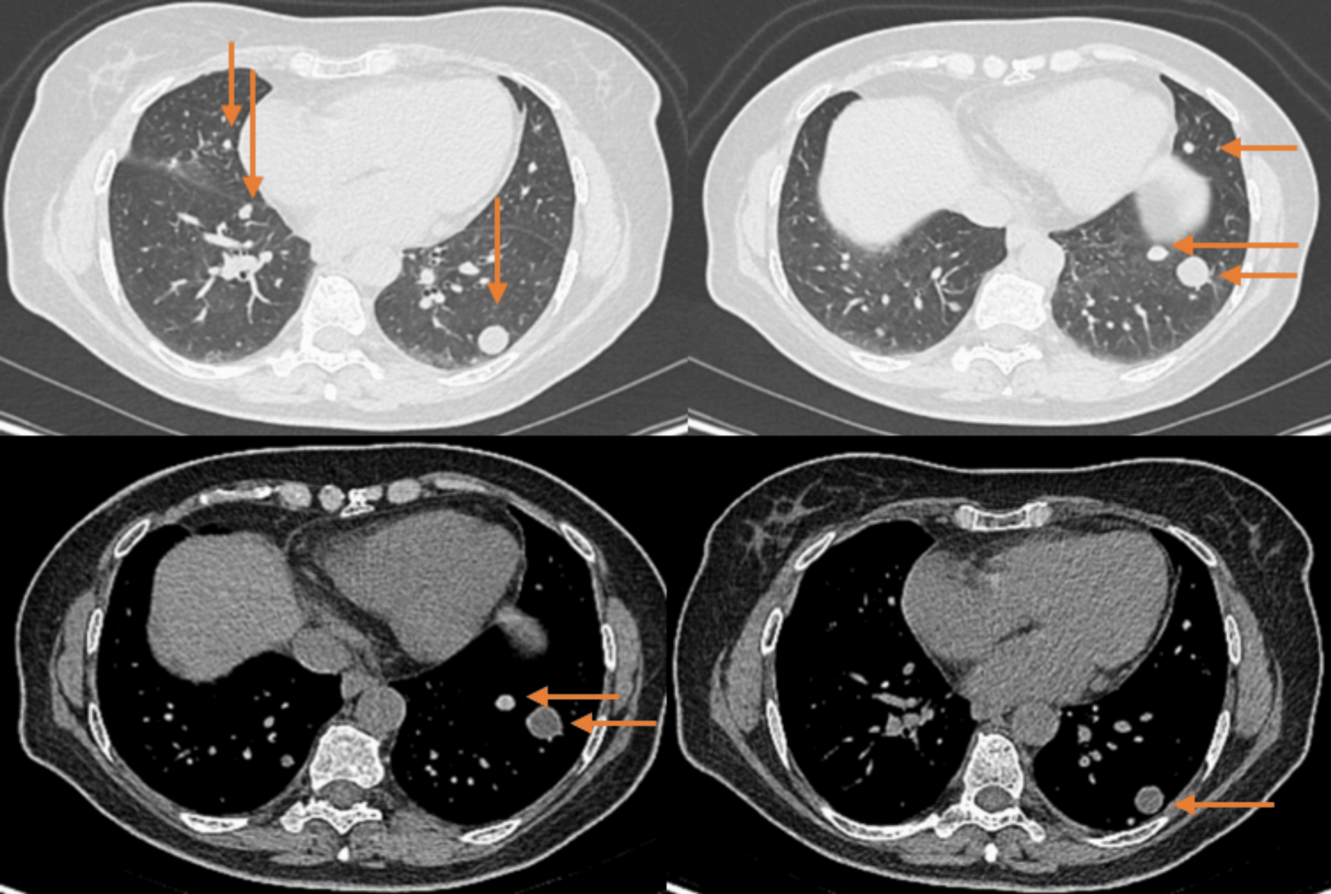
CT images show multiple, well-demarcated, round lung nodules of various sizes (orange arrows), two years ago
